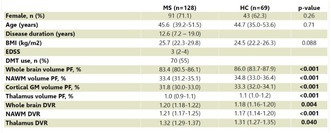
TABLE 1 Clinical characteristics, MRI volumes, and TSPO Distribution Volume Ratios (DVR) for MS patients and healthy controls. Expressed as median (interquartile range) unless otherwise stated.
